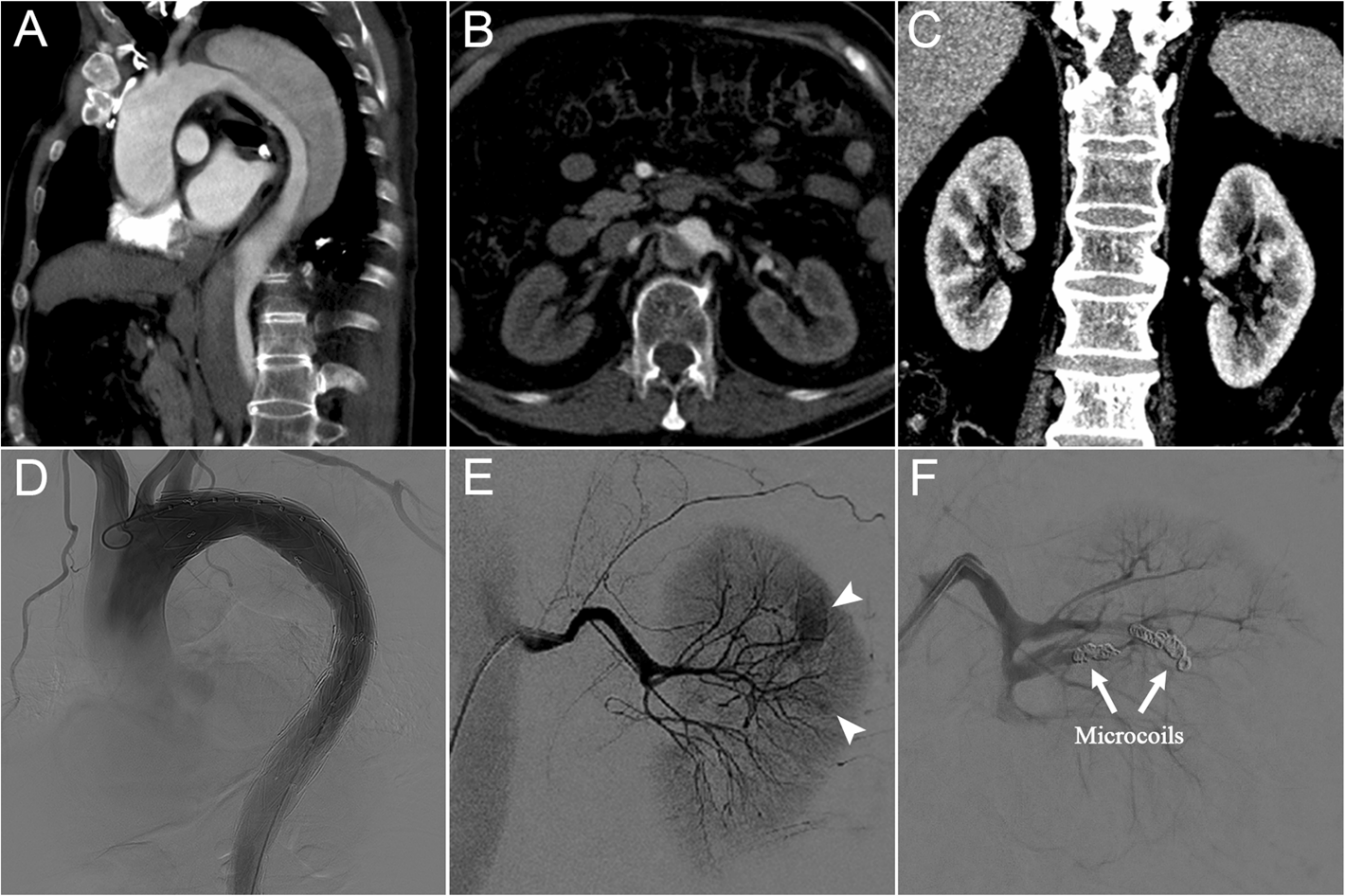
(a) Preoperative sagittal computed tomography angiography showed a type B dissection ranging from the distal aortic arch to the iliac arteries. (b) The left renal artery originated from the true lumen, and (c) without cysts or tumors. (d) Completion angiography showed the entry tear was successfully occluded. (e) Left renal angiography revealed two active bleeding sits located in the distal branches of left renal artery (arrowheads). (f) Completion angiography showed the effective occlusion of the feeding vessel and termination of the left renal bleeding
